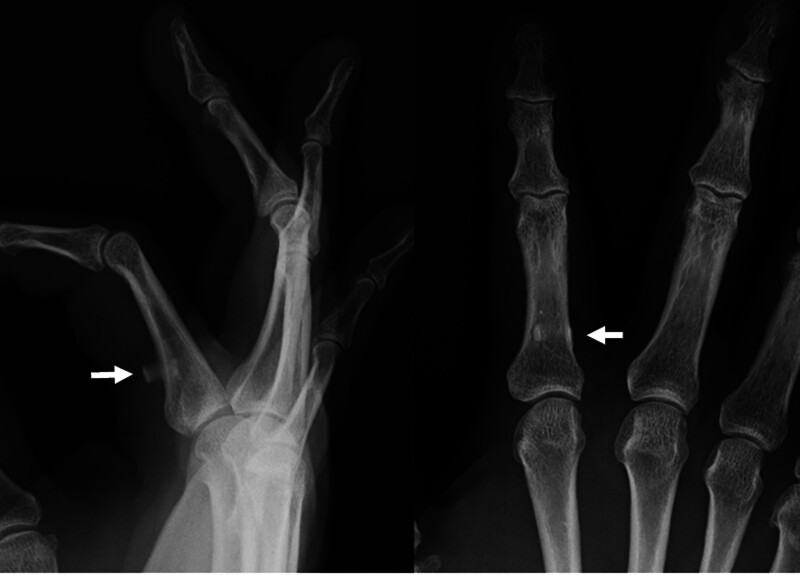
Radiographs of the right hand. Arrows indicate heterotopic ossification.
